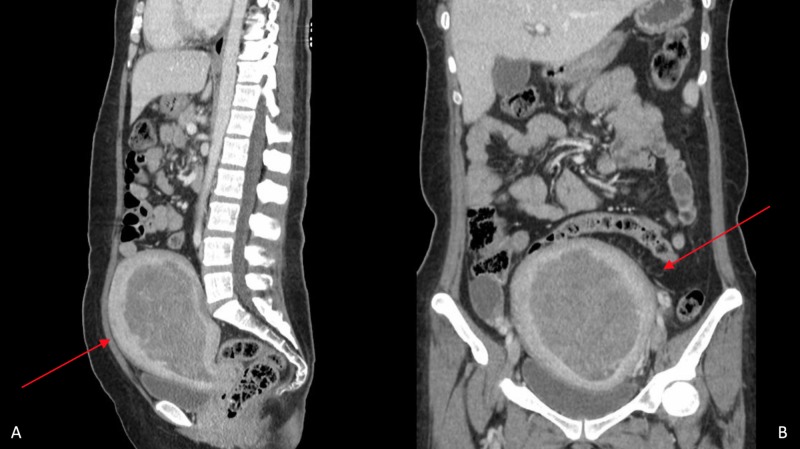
Sagittal and coronal views of the CT scan of the abdomen/pelvis with contrast Panes A (sagittal) and B (coronal) reveal diffuse distention and heterogeneous attenuation centered within the endometrium with diffuse enlargement (15 x 13.5 x13 cm) of the uterus (red arrows).
